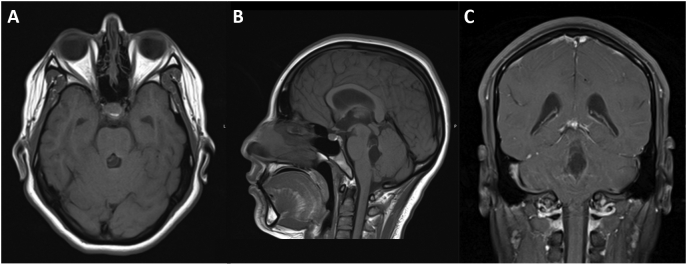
Preoperative Magnetic Resonance Imaging (MRI) scans showing racemose neurocysticercosis (NCC) lesion. (A) Axial pre-contrast MRI T1 scan. (B) Sagittal pre-contrast MRI scan. (C) Coronal post-contrast MRI scan.
